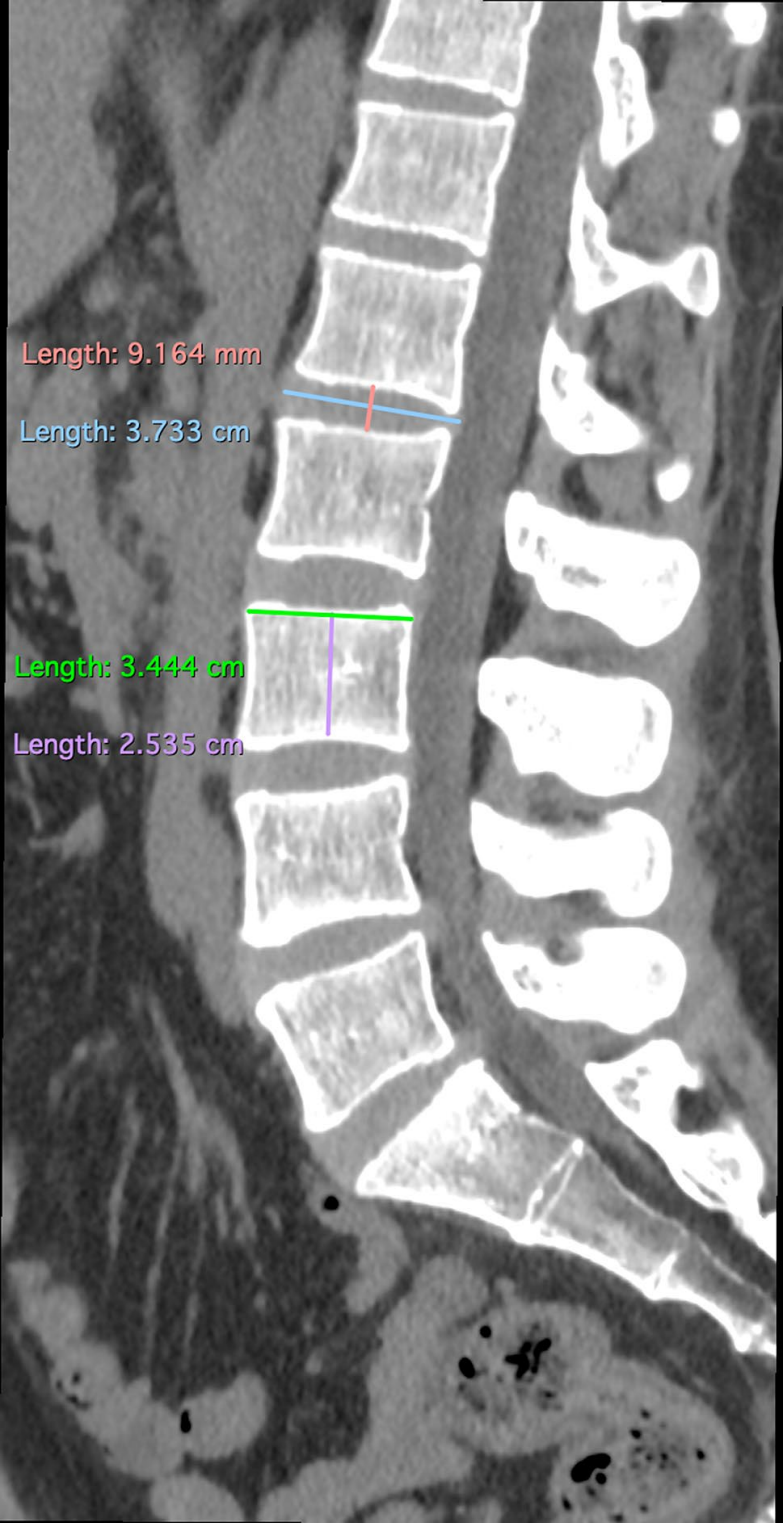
Lumbar spine computed tomography (sagittal) measurements of vertebra’s and disc’s height and length
